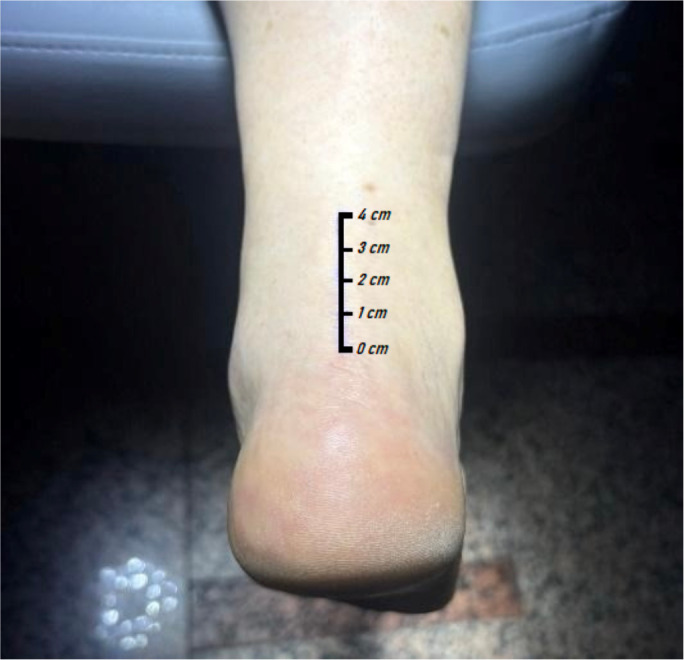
Ultrasound measurements performed 4cm proximal to the Achilles tendon insertion and at the mid-portion of the tendon.
